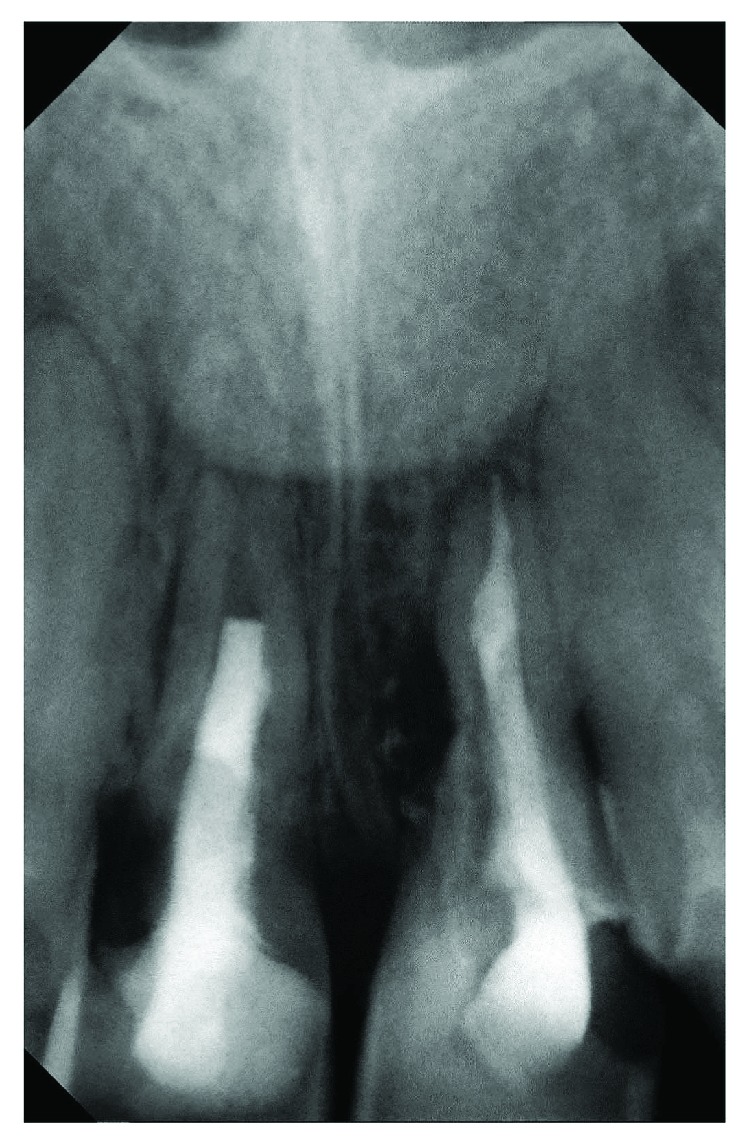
X-ray presenting severe root resorption of teeth 11 and 21.
